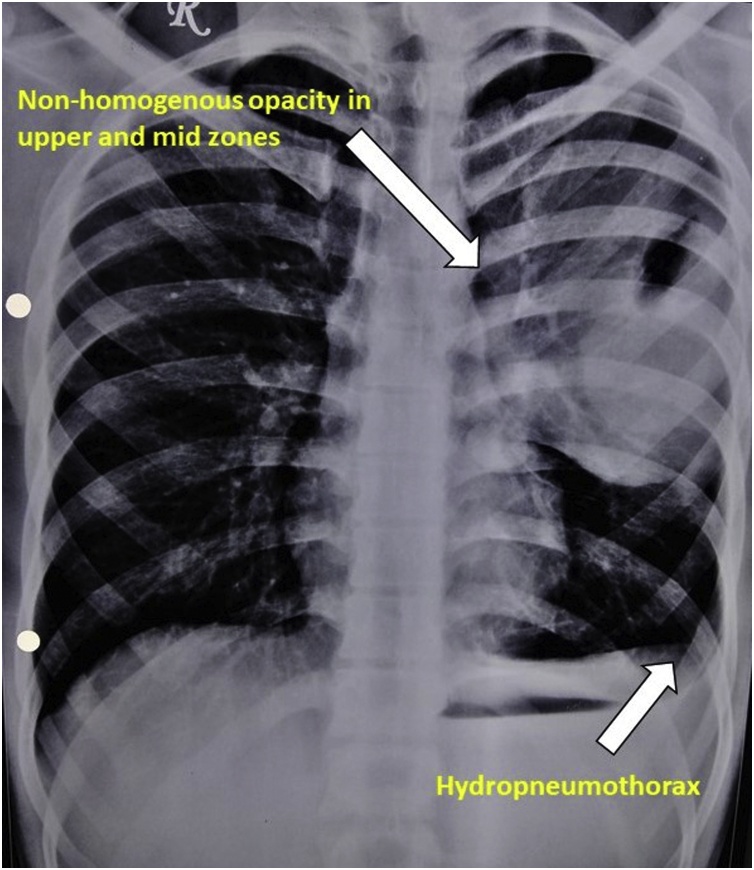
Chest radiography was done suggestive of “Non-homogenous opacity in the left upper and mid zones with left sided hydropneumothorax”.
